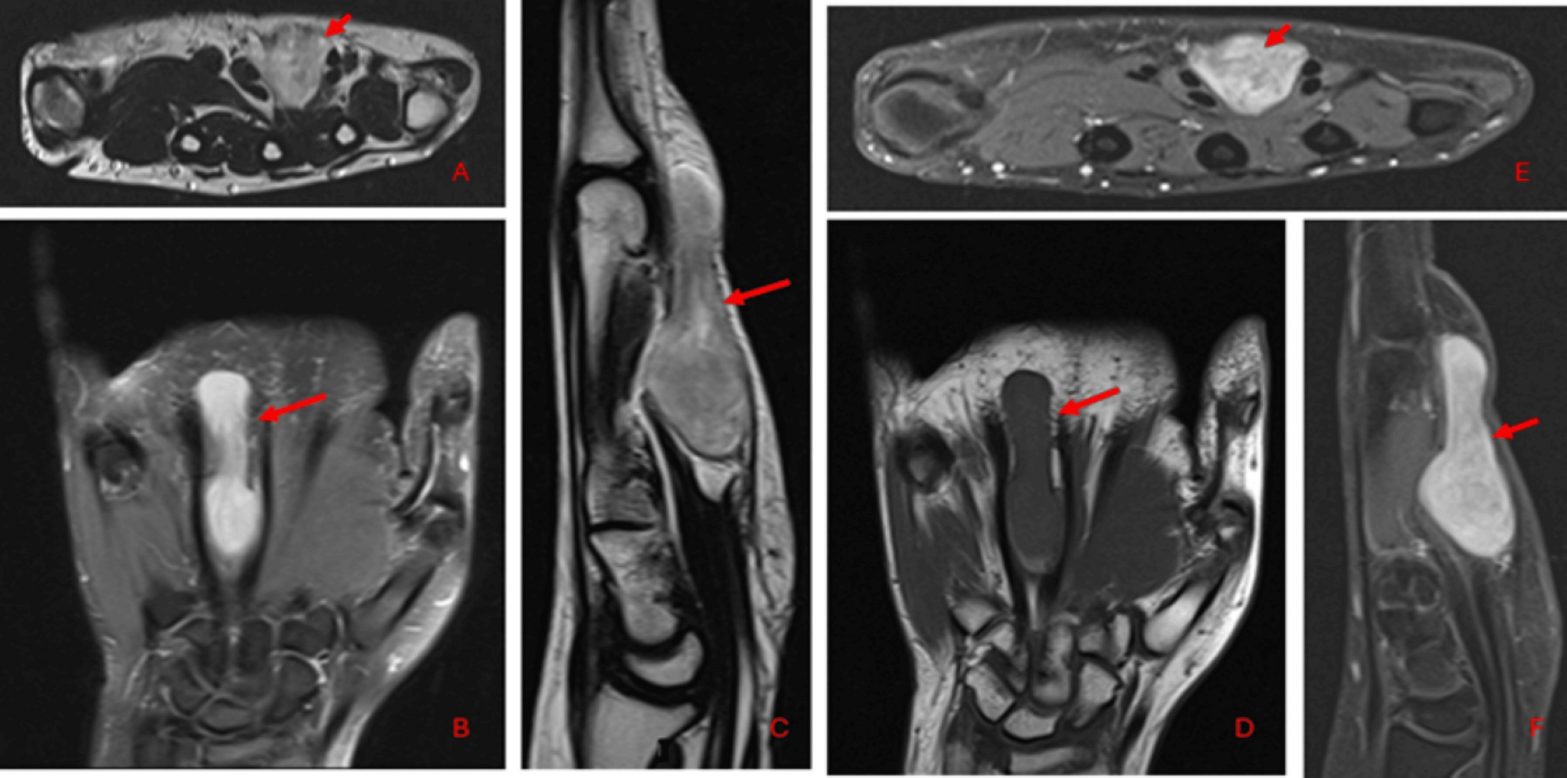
Preoperative MRI of the hand showing a lobulated mass within the intercarpal space between the third and fourth flexor tendons and lumbricals. It demonstrates hyperintense signals (red arrows) on axial T2WI (A), coronal PD (B), and sagittal T2WI (C) images and hypointense signals on coronal T1WI images (D). Intense enhancement on axial (E) and sagittal (F) post-IV gadolinium contrast images are also observed MRI: magnetic resonance imaging; T2WI: T2-weighted imaging; PD: proton density; T1WI: T1-weighted imaging; IV: intravenous
